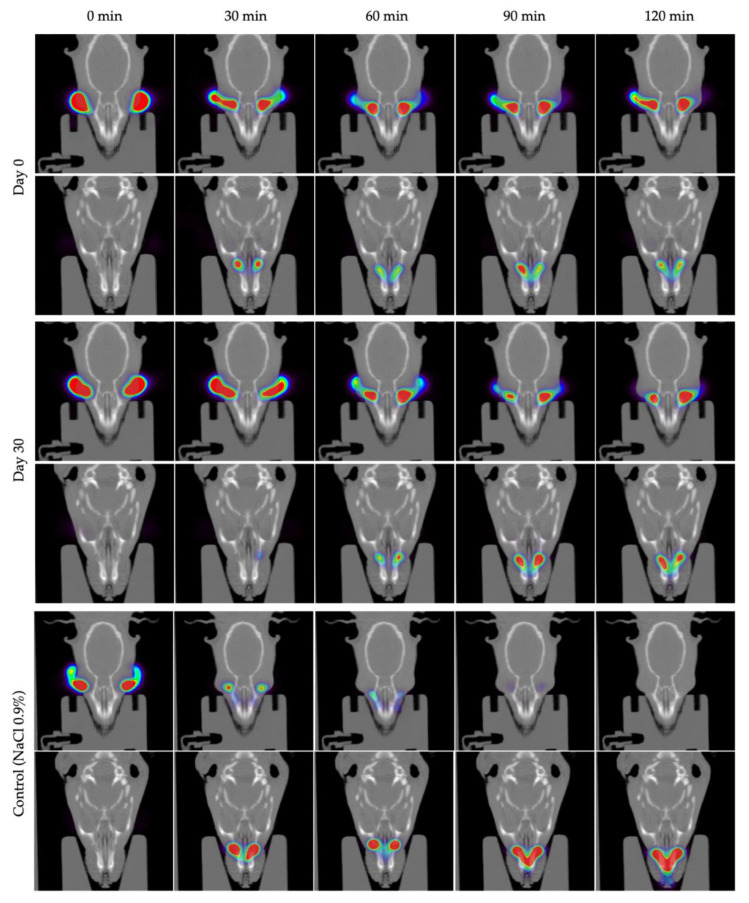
Positron emission tomography (PET) images (maximum intensity projections, coronal views) obtained at different time points after ocular administration of eye drops labelled with ^18^F-fluorodeoxyglucose (^18^F-FDG).
